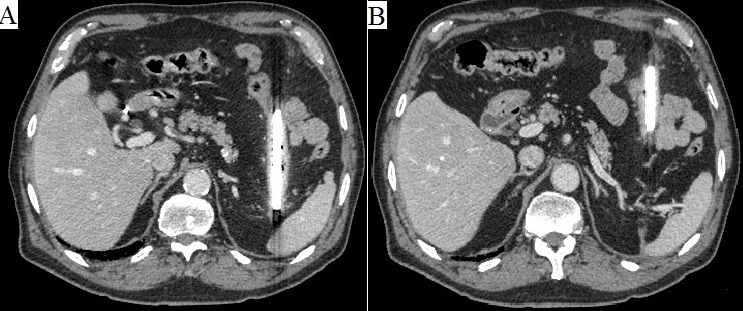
(A) abdominal CT scan: posterior end of the shard next to the spleen; (B) arrow points at the retroperitoneum scar, where the glass shard impaled the patient
